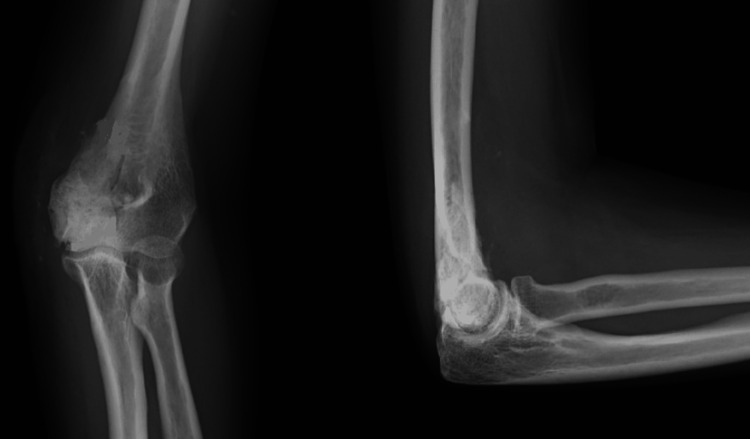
Post-operative X-ray at the end of two years Anteroposterior and lateral views of the right elbow at the end of two years
